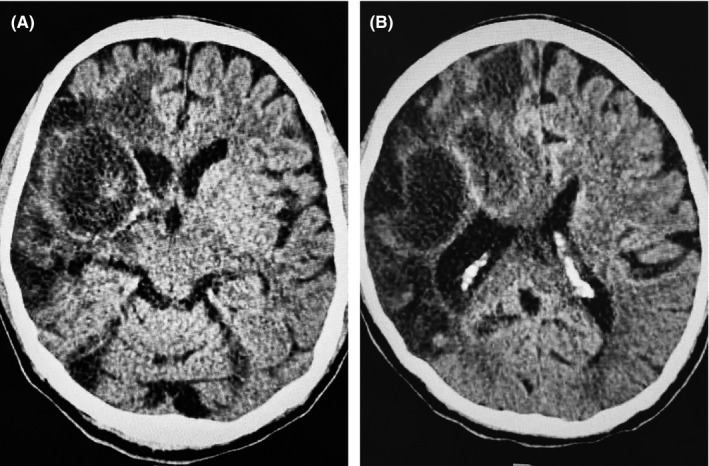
A, B CT with contrast images
